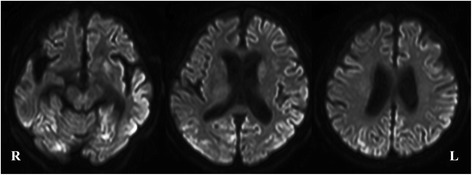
Head MRI (axial, diffusion-weighted image) of the patient. Diffusion-weighted brain images showed high intensity lesions, separately in the cortex of the left temporo-parietal lobe, right parietal lobe, left frontal lobe, left insula, and left basal ganglia
